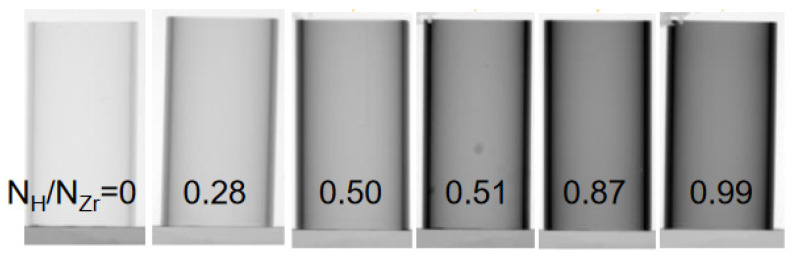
A calibration sample set of Zircaloy-4 (Zry-4) cladding tube samples with heights of 20 mm, where the neutron attenuation increases with an increasing amount of hydrogen in the metal. The neutron radiographs were taken at the ICON beamline at the PSI.
